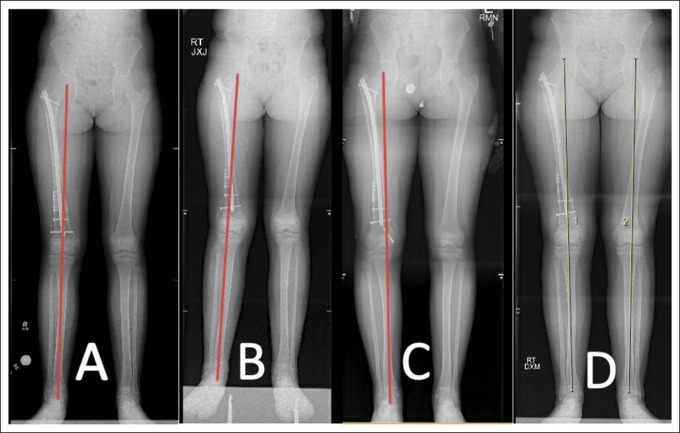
A, Initial postoperative radiograph with a neutral mechanical axis. B, Radiograph showing right distal femoral valgus noted 16 months after the index procedure. C, Radiograph showing correction with medial hemiepiphysiodesis of the distal femur, obtained 3 months postoperatively. D, Radiograph showing the maintained mechanical axis 1 year later. Transphyseal screw removed.
